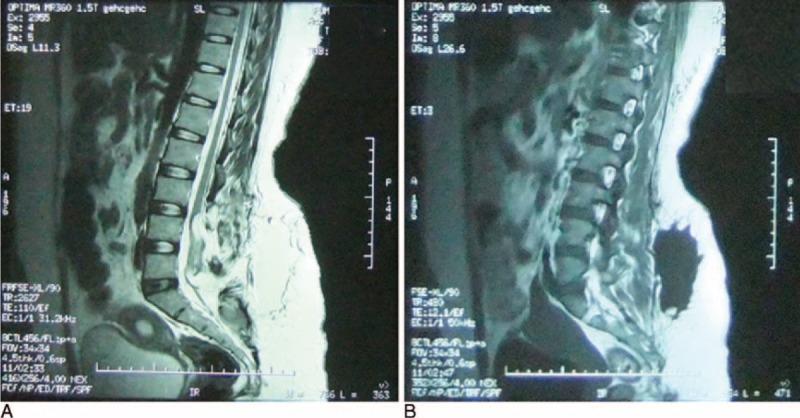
Preoperative MRI sagittal views of the lumbosacral region on postoperative day 6. A massive, irregular subcutaneous watery area with (A) long T2 and (B) long T1 signals at the L4 to S2 levels is observed. MRI = magnetic resonance imaging.
